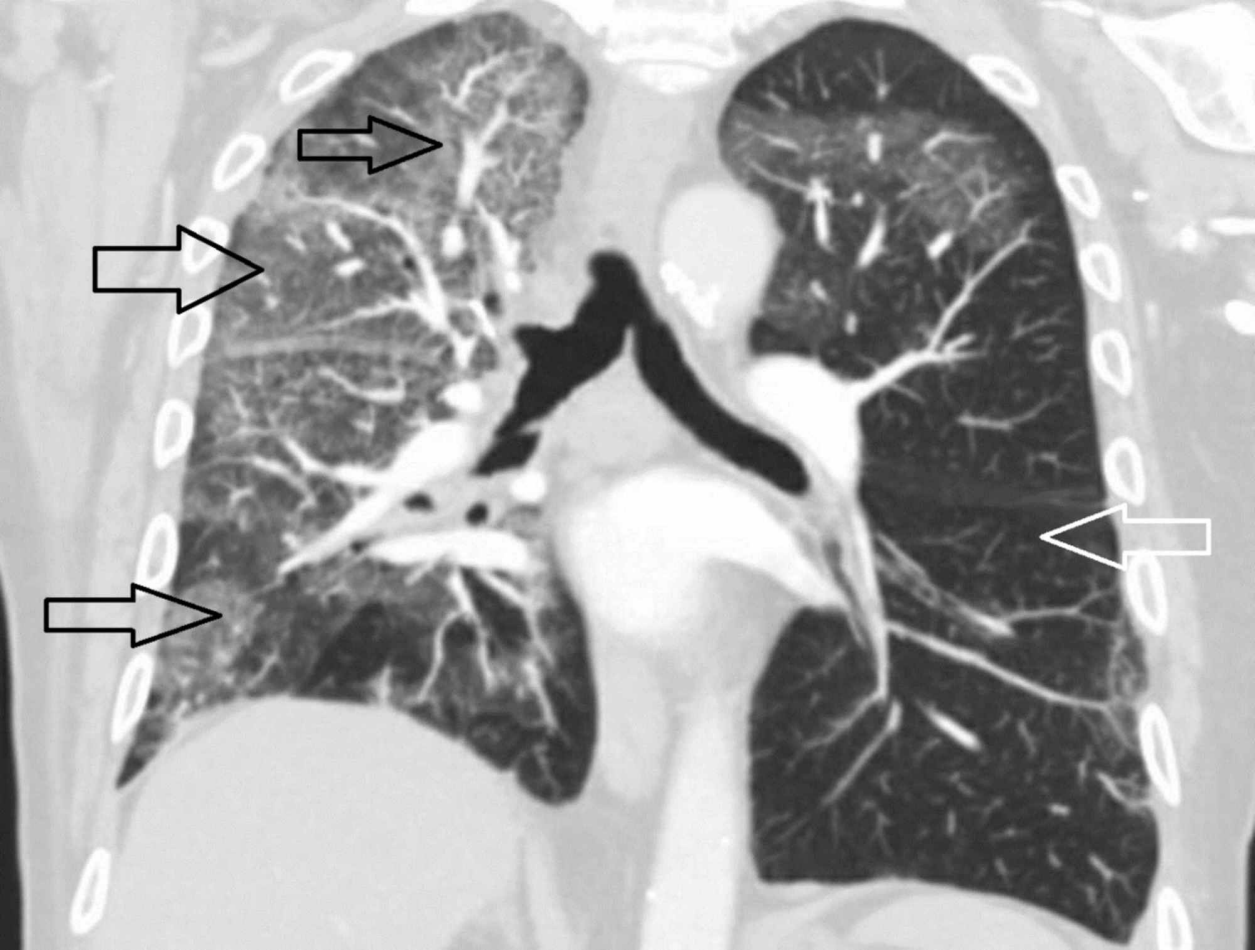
Coronal view of CT scan of thorax demonstrating extensive parenchymal lung disease involving all three lung lobes (black arrows). Left lung is relatively normal (white arrow).
